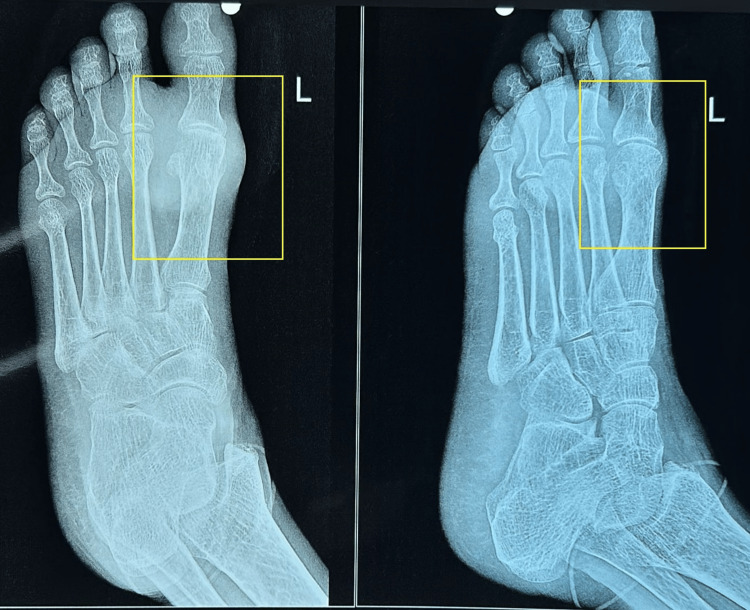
X-ray imaging of the left foot over the dorsal aspect and anteroposterior plane
